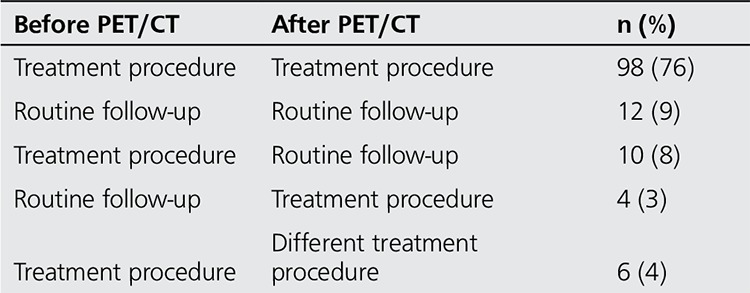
Impact of PET/CT in clinical decision making
